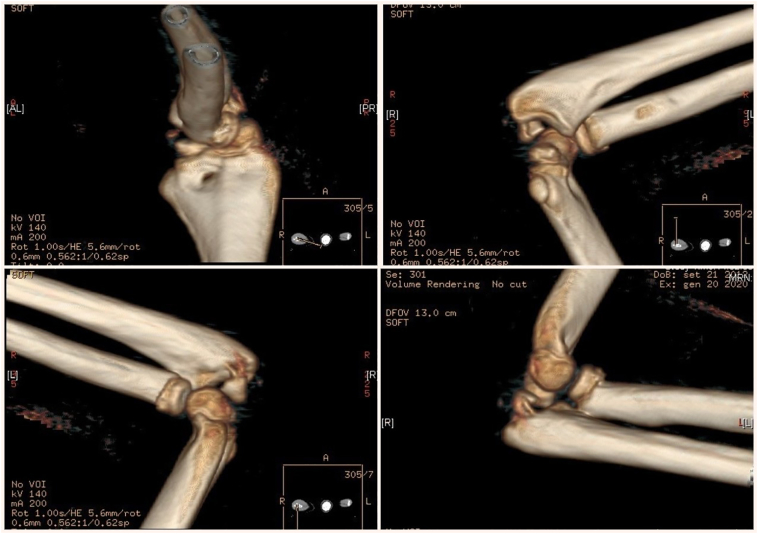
Pre-operative CT – scan with 3D reconstruction confirms an clarifies trans-olecranon fracture dislocation of the elbow associated with a radial head fracture.
